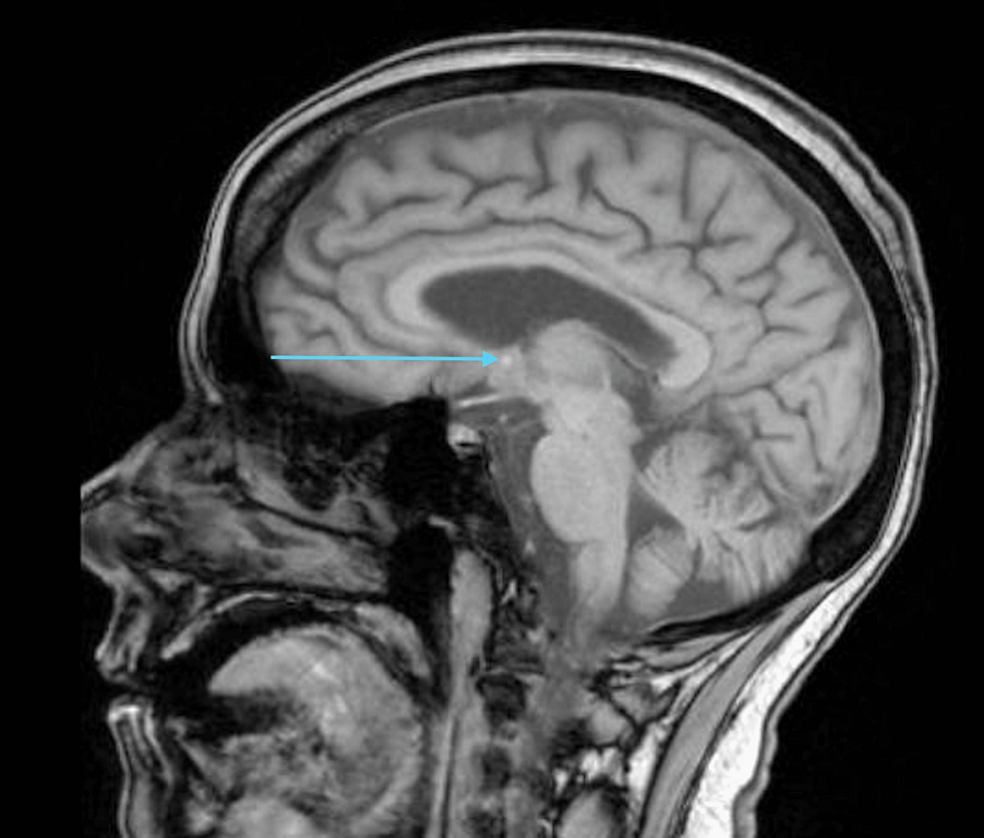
Sagittal T1 MRI brain and pituitary gland with hyperintense signal concerning for an ectopic posterior pituitary gland Blue arrow: Hyperintense signal concerning for posterior pituitary gland
